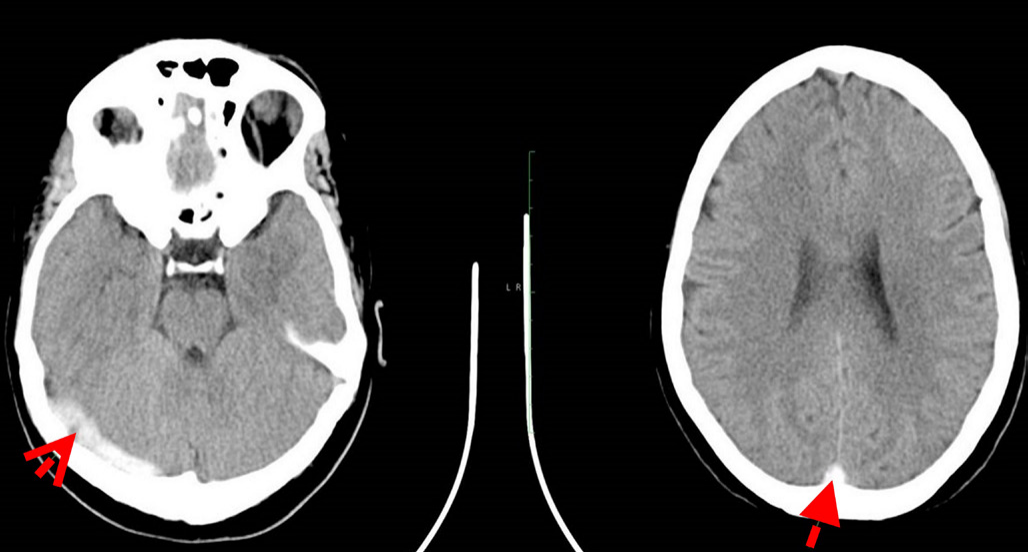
Brain CT findings on admission. The first red arrow shows a left parasagittal parietal lobe high convexity gyral hypodensity (1.8×0.9 cm) region is seen. Appearances may be caused by a venous infarct. The second red arrow shows an abnormal superior sagittal and left sigmoid sinus hyperdensity suspicious for venous sinus thrombosis (empty delta sign). Relative hypodensity in the left internal capsule. No other areas of abnormal attenuation. Otherwise normal appearances of the brain parenchyma, ventricles, cisterns and nuclei. No extra-axial collections. No intraparenchymal haemorrhage detected. Impression: left parietal lobe venous infarct. Venous dural sinus thrombosis.
